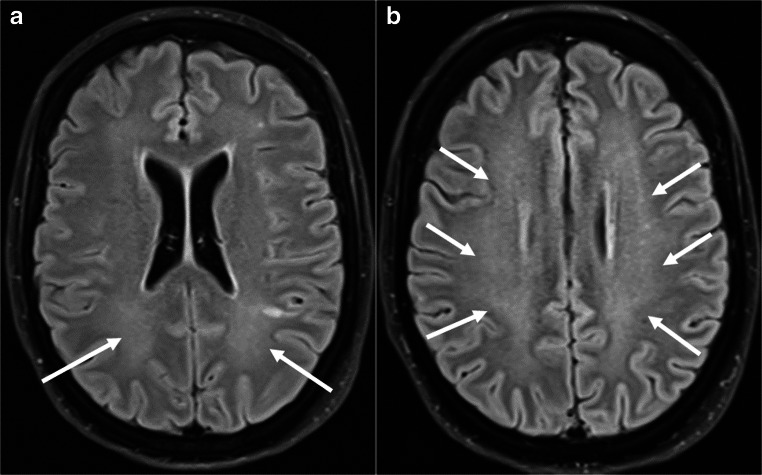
a, b A 44-year-old female patient with COVID-19 and severe ARDS. Axial FLAIR MR images show bilateral, ill-defined hyperintensity of the white matter consistent with leukoencephalopathy (white arrows)
